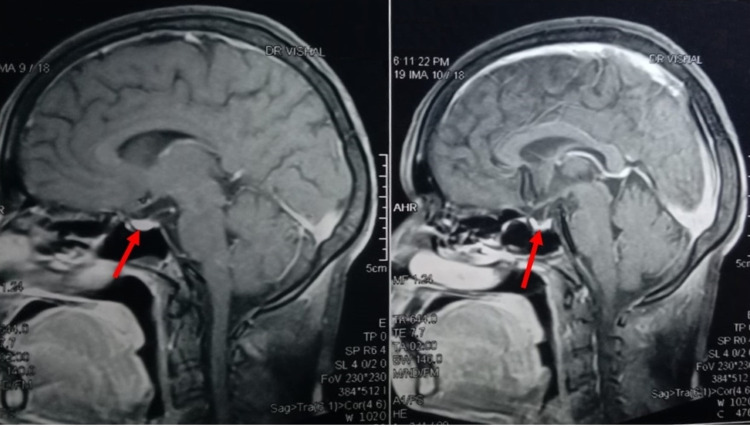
MRI of the mid-sagittal section of the brain showing partially empty sella turcica with a normal stalk (arrows).
